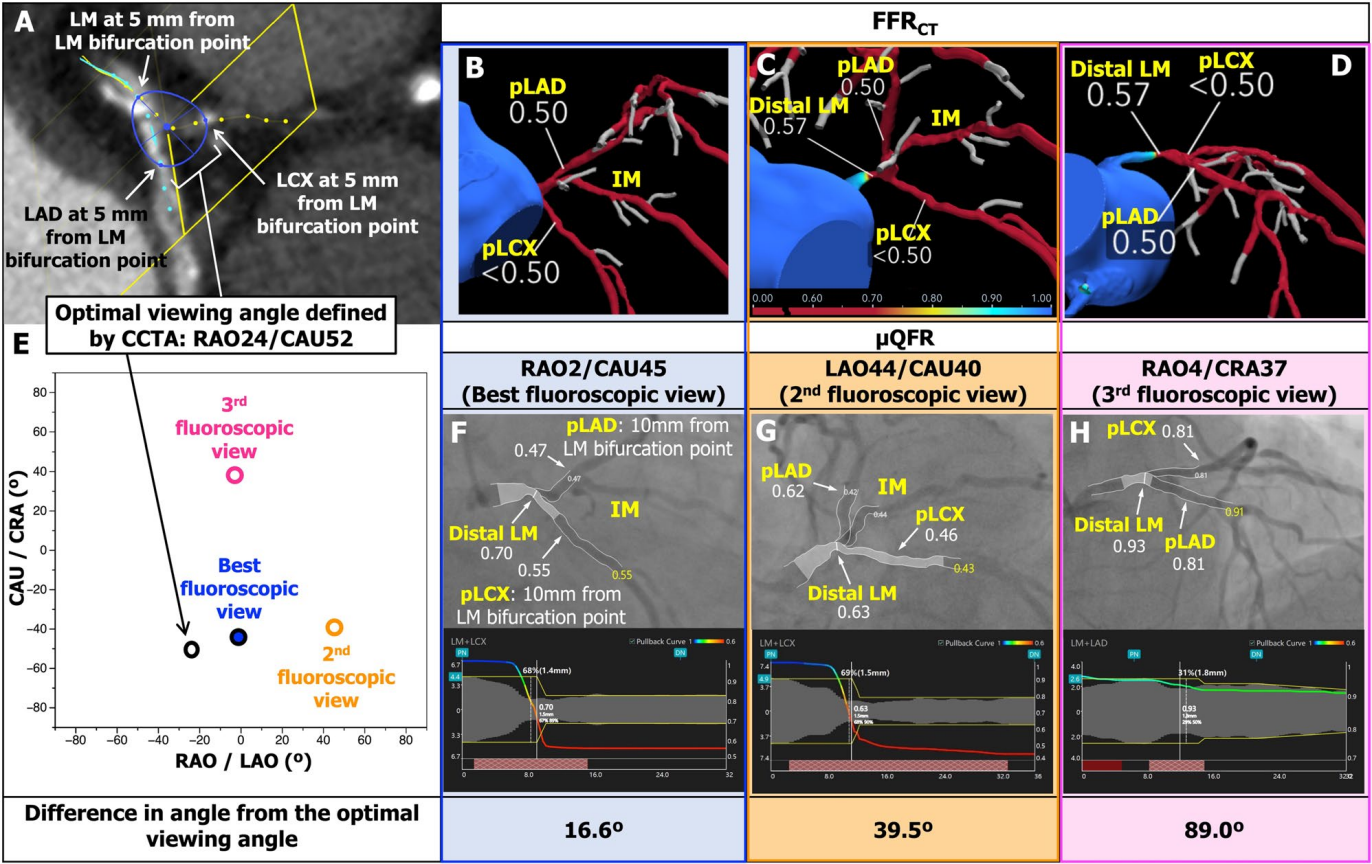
Example of image analyses of CCTA (A), FFR_CT_ (B–D), and µQFR (E–H). The optimal viewing angle of LM bifurcation was defined on CCTA analysis (A). The best fluoroscopic view was defined as the closest X-ray gantry angulation to the optimal angle defined by CCTA (E). Matched views of the FFR_CT_ and angiography by µQFR were presented in panels B–D and F–H. *CAU* caudal, *CCTA* coronary computed tomographic angiography, *CRA* cranial, *FFR*_*CT*_ fractional flow reserve derived from computed tomography, *LAO* left anterior oblique, *LM* left main coronary artery, *pLAD* proximal left anterior descending artery 10 mm distal to the LM bifurcation point, *pLCX* proximal left circumflex artery 10 mm distal to the LM bifurcation point, *RAO* right anterior oblique, *µQFR* Murray law-based quantitative flow reserve ratio
